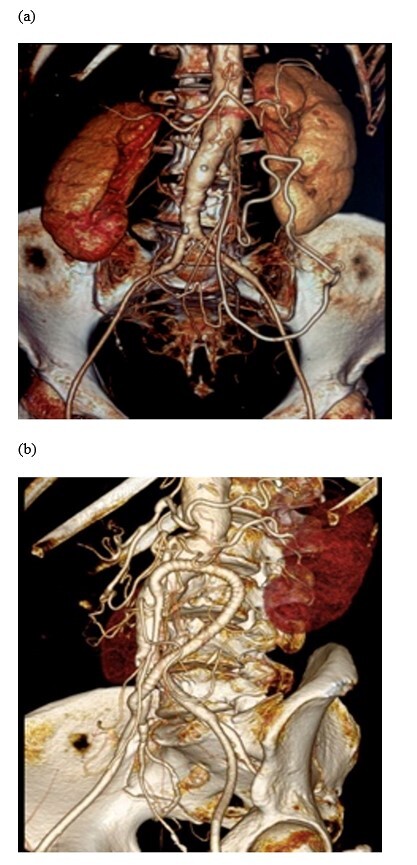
Intraoperative and imaging findings for patient #3. (a) Preoperative computed tomography angiography (CTA) with celiac trunk stenosis. The superior mesenteric artery (SMA) and the inferior mesenteric artery are occluded; (b) Postoperative CTA with a patent reinforced PTFE graft from the right iliac artery to the SMA.
